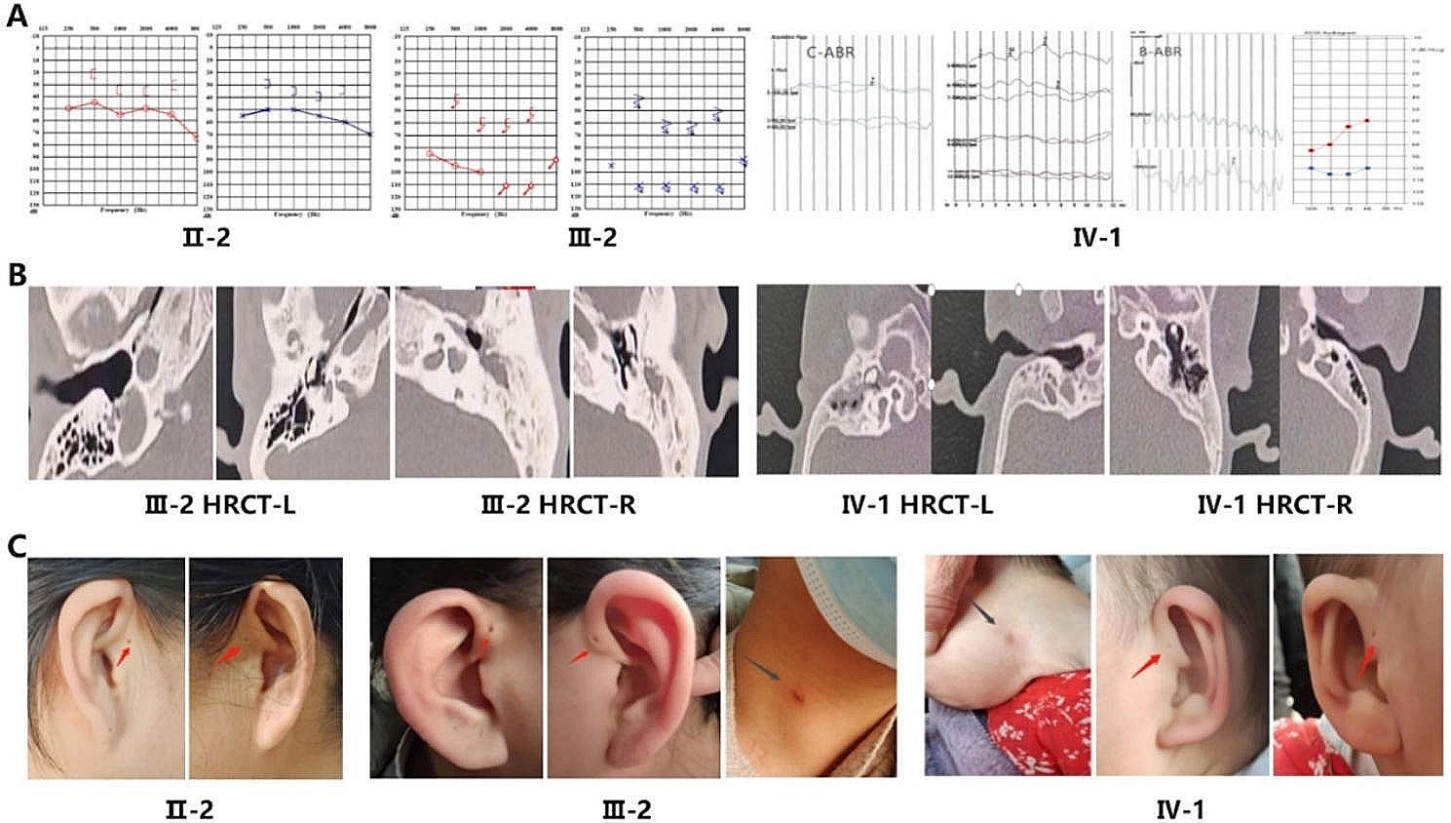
Audiograms, CT images of the temporal bone and clinical features. (A) pure-tone audiometry, auditory brainstem response or auditory steady-state response; (B) Axial CT images of the temporal bone from subject III-2 and IV-1; (C) medical photographs of patients: Black arrow: branchial cleft fistulae or scar; red arrow: preauricular pits
